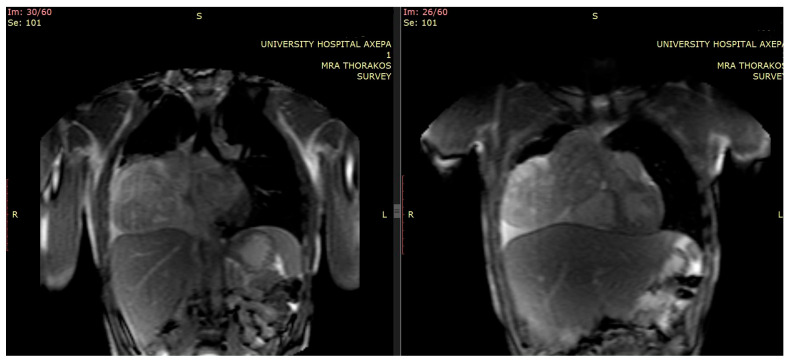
Initial preoperative MRI.
